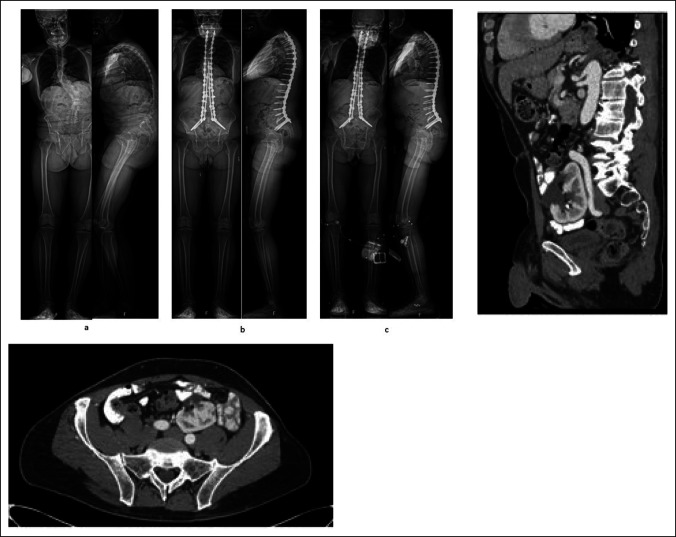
Top Left: AP and lateral full-length standing radiographs (A) preoperatively, (B) 1 month postoperatively, and (C) 20 months postoperatively. Top Right: Preoperative sagittal CT scan demonstrating pelvic kidney and vascular anatomy precluding anterior retroperitoneal approach to the lumbar spine. Bottom: Preoperative axial CT scan demonstrating pelvic kidney and vascular anatomy precluding the anterior retroperitoneal approach to the lumbar spine.
